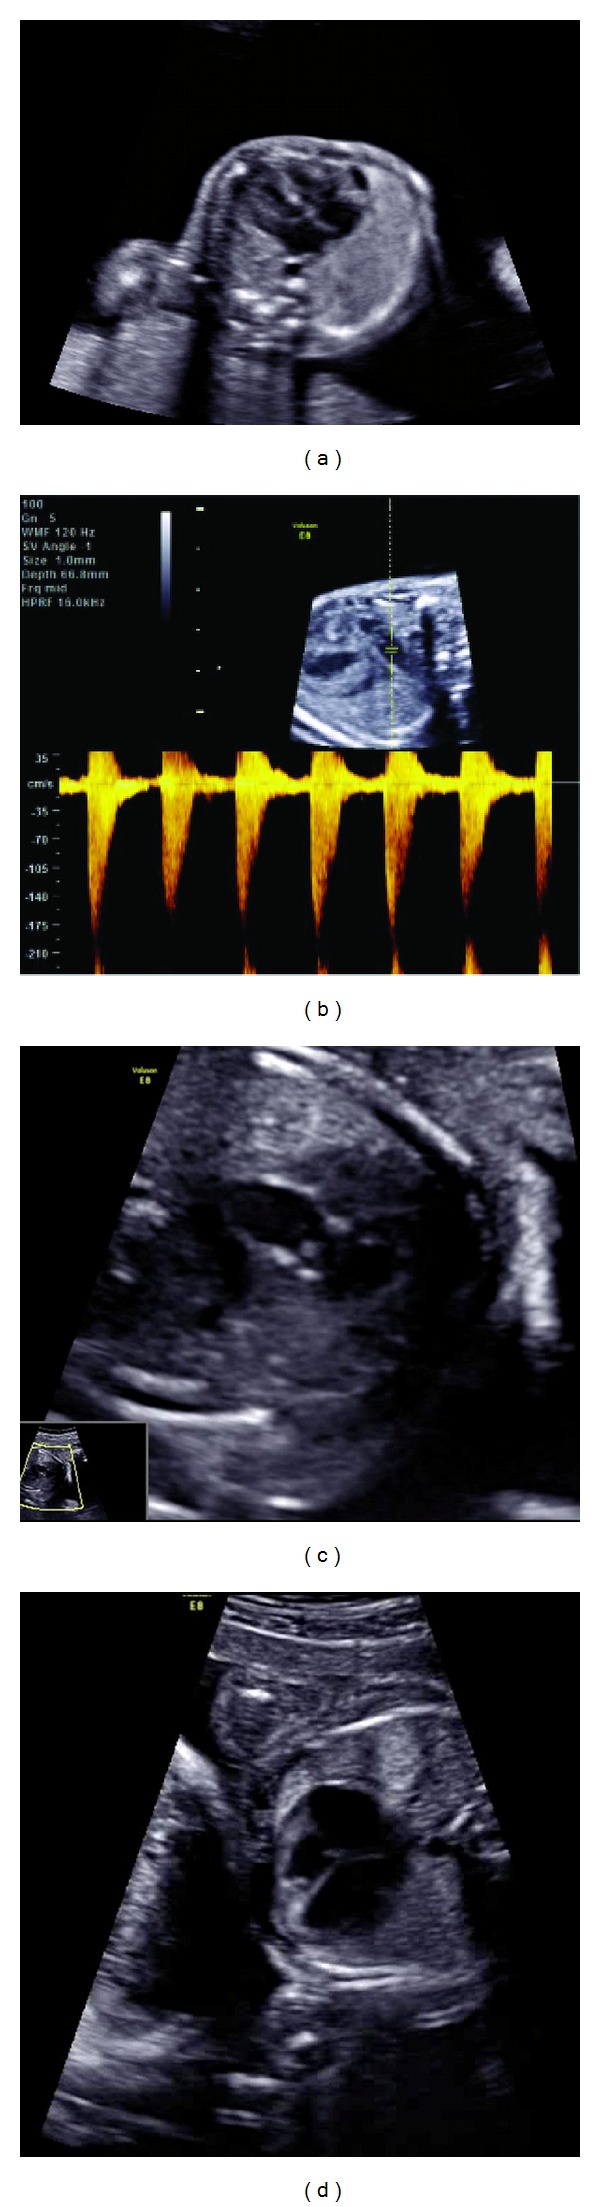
(a) Ultrasound examination at 21 weeks GA shows a four chamber view with a very mild right ventricle hypertrophy and mildly pericardial effusion. (b) Ultrasound examination at 21 weeks GA shows an elevated peak systolic velocity across the pulmonary valve of 1.75 m/s. (c) Ultrasound examination at 21 weeks GA shows the pulmonary valve with a mild post stenotic dilatation. Valve cusps and pulmonary wall were echogenic and thickened. (d) Ultrasound examination at 28 weeks GA shows RV remains adequate, mild hypertrophy.
